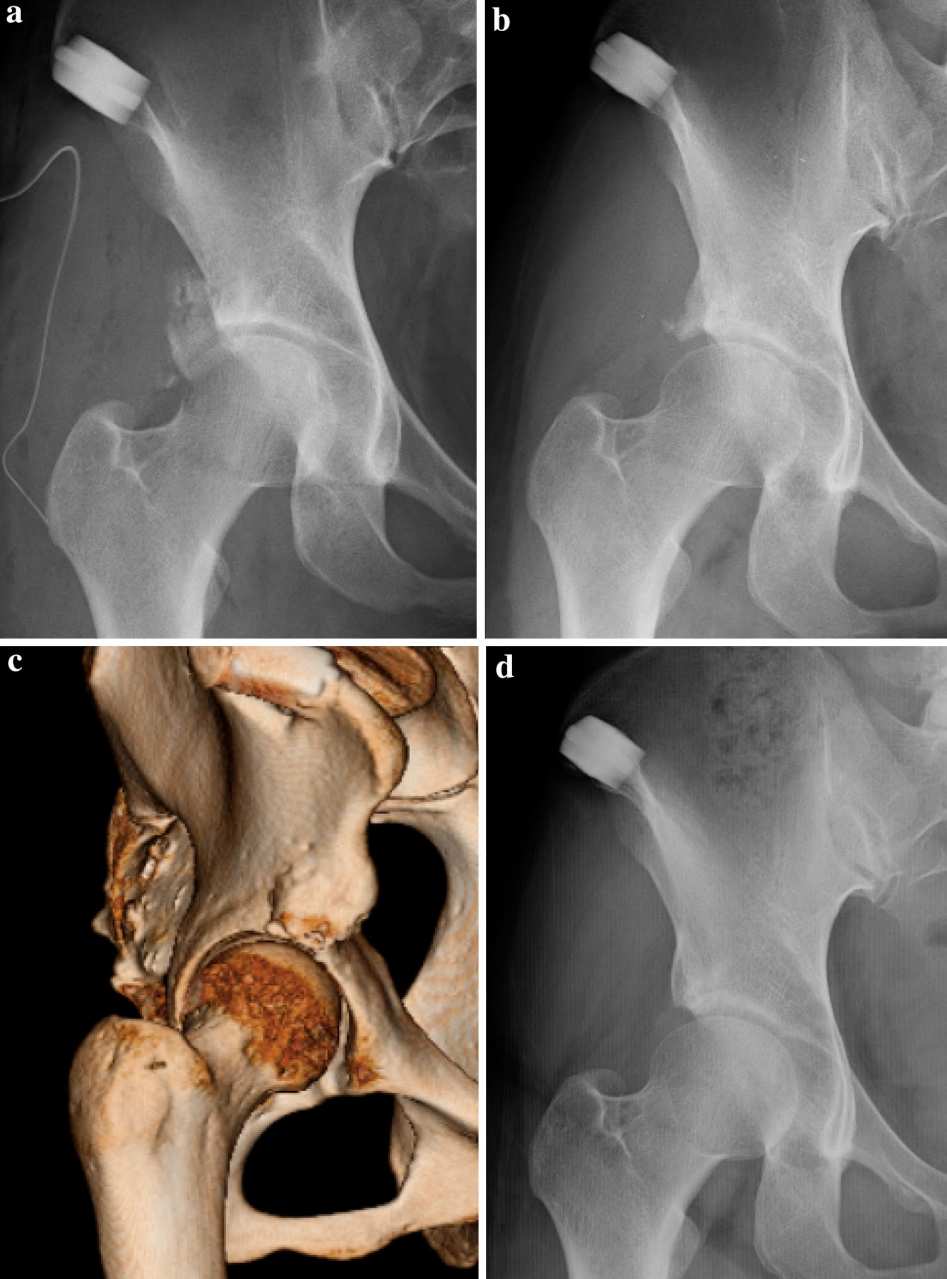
a Pelvic AP radiograph showing improved coverage of the acetabulum with a shelf graft just after surgery. LCE angle was 41°. b Pelvic AP radiograph showing proper resorption and remodeling of the shelf graft at 1 year after surgery. c 3D image of CT showed the location of the shelf graft at 1 year after surgery. d At 4 years postoperative, a pelvic AP radiograph shows a LCE angle of 34°
